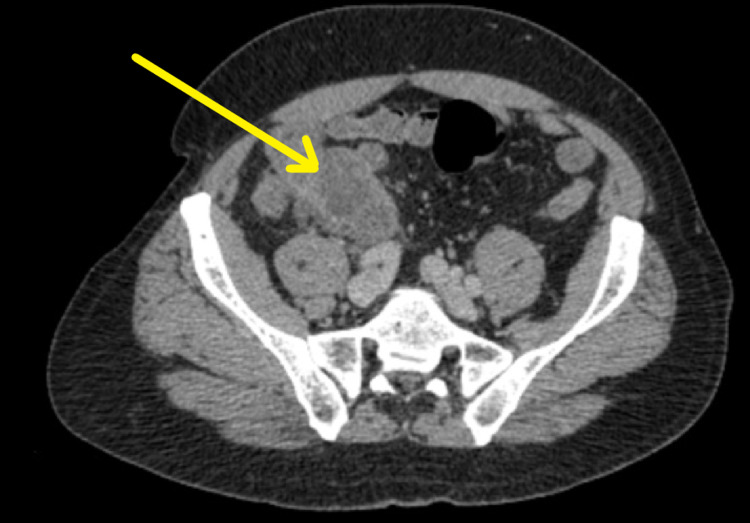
CTAP on admission CTAP: CT abdomen and pelvis
